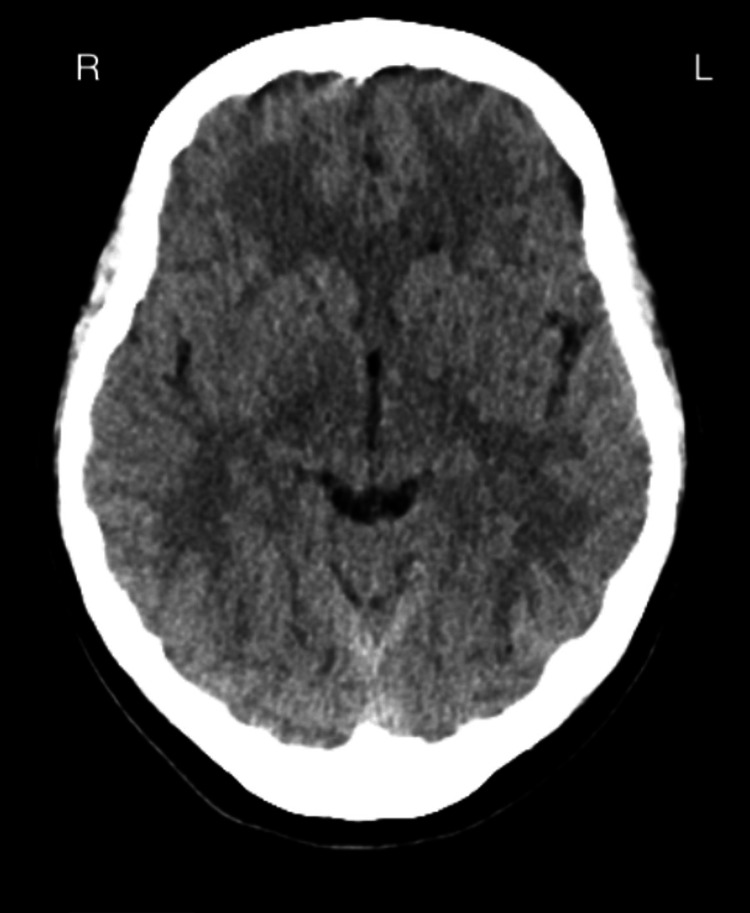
Non-contrast head CT showing no acute intracranial abnormalities. Diffusely hyperdense vascular structures are suggestive of dehydration.
